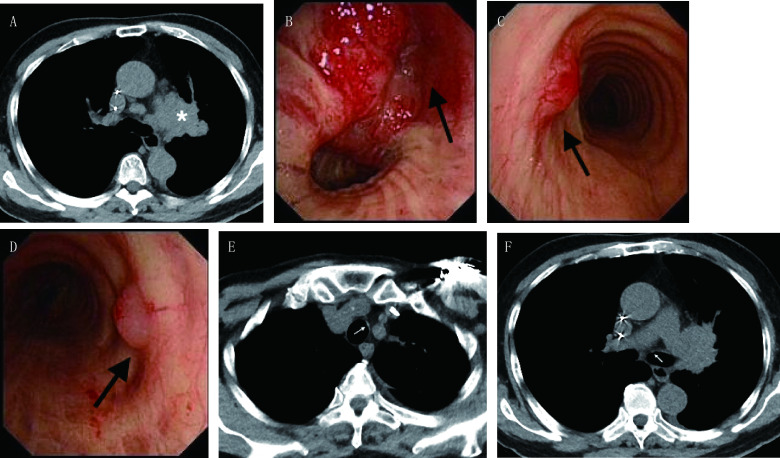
小细胞肺癌患者发生EEM的胸部影像和支气管镜表现（表 1中患者6）。A：CT示左肺门肿块（星号）；B、C、D：支气管镜示左肺上叶可见新生物致管腔完全闭塞（B箭头），气管上段左外侧壁息肉样病变（C箭头），左主支气管入口左侧壁息肉样病变（D箭头）；E、F：回顾肺CT分别可见气管和左主支气管局部管壁增厚（箭头） Chest radiographic and bronchoscopic images in a patient with SCLC and EEM (patient 6 in Tab 1). A: Chest CT showing an irregular mass in the left perihilar lung; B, C, D: Bronchoscopy revealing the left upper lobe bronchus was occluded completely by an endobronchial neoplasm (B, arrow), one polypoid lesion located in the left lateral wall of the upper trachea (C, arrow), and the other polypoid lesion in the medial wall of the left main bronchus inlet (D, arrow); E, F: Review of his chest CT showing eccentric thickening of the upper trachea (E, arrow) and the left main bronchus (F, arrow)
